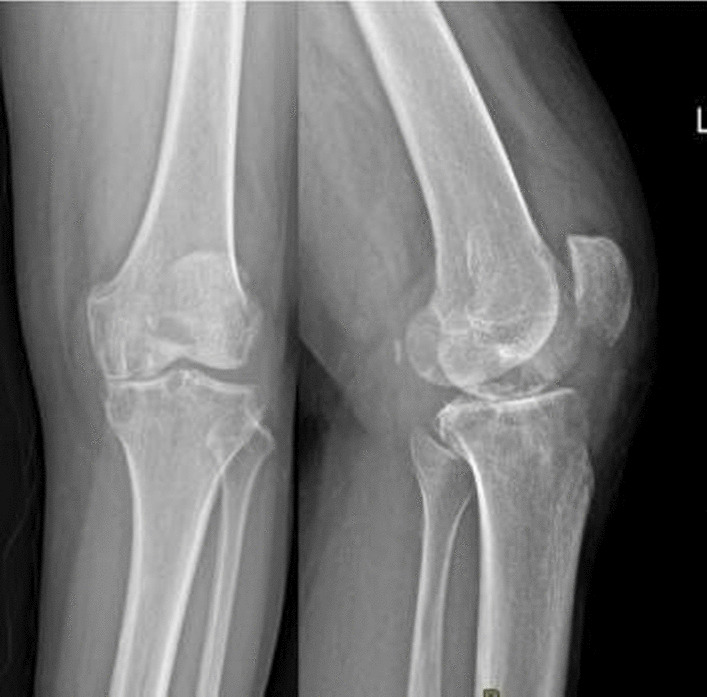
X-ray showing obvious cystic changes in the proximal tibia and distal femur of the left knee joint
